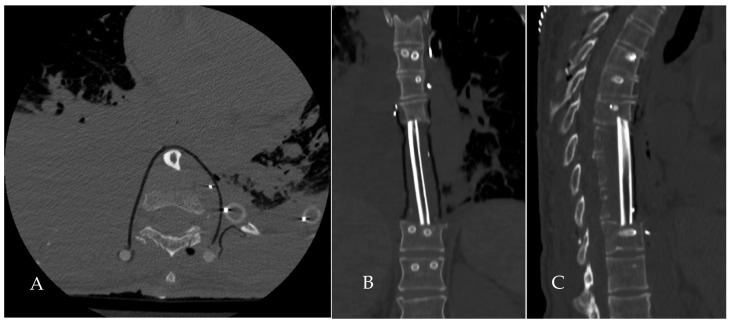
Post-operative CT scan image at the lower T9 level, distal to the sagittal leg of the osteotomy. As planned, the coronal osteotomy spared the circumference of the spinal canal and runs some millimeters from the tumor posterior margin. A Gore-Tex sheet is left, creating a new compartment from the mediastinum. Panel (A), axial section. Panels (B,C), coronal and sagittal sections showing the grafted fibula.
